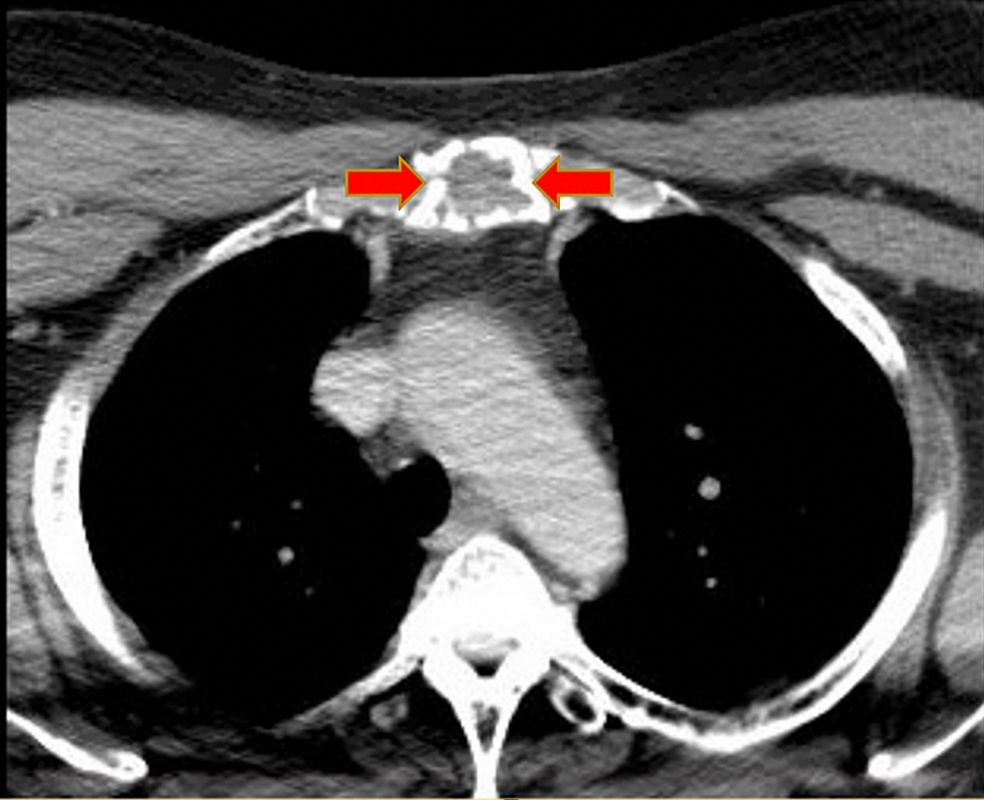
CT scan showing incompletely visualized expansile mass of the manubrium/upper sternum with irregularity and erosion of the cortex (red arrows)
